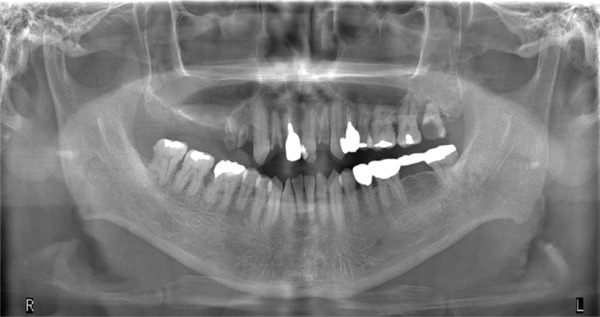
Panoramic radiograph showing no bone resorption.
